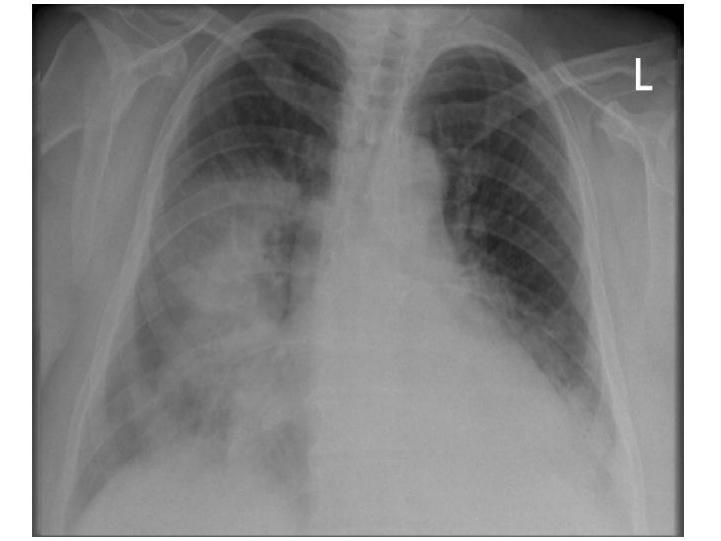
Chest X-ray (PA view) at initial presentation showing consolidation within the right mid and both lower lobes.
